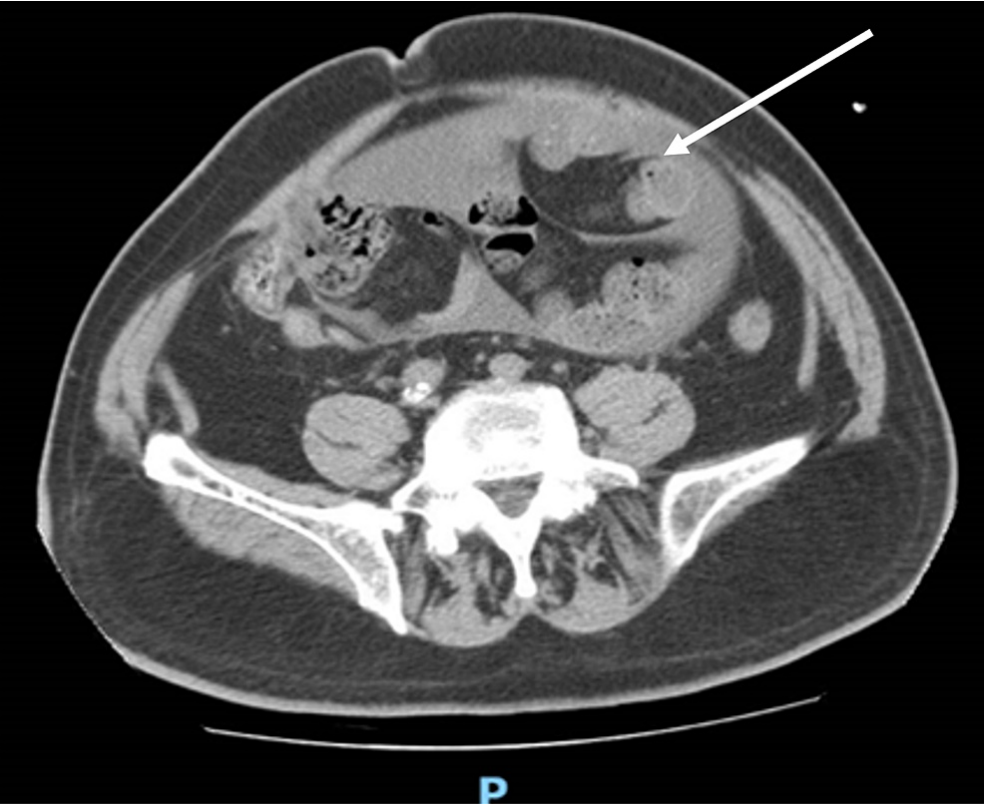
Coronal sections of abdominal CT images indicate small bowel dilation with fluid involving the bowel loops.
